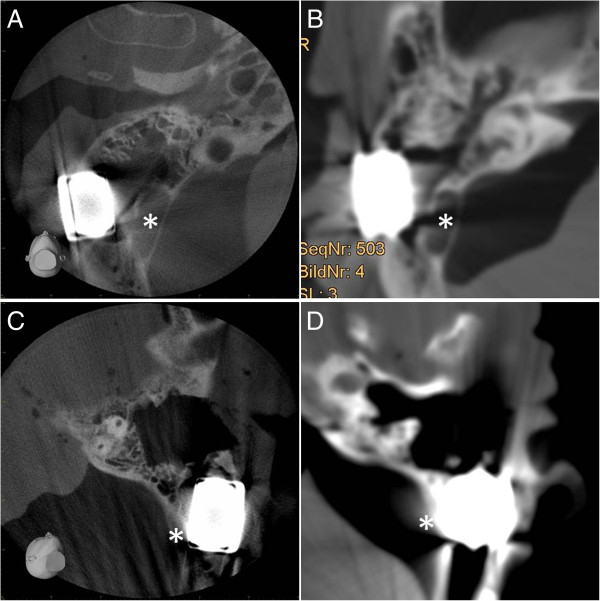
Positional relation of the implant to the sinus sigmoideus. Visualisation of the implants in CBCT (A and C) and in CT (B and D) for both prepared skulls (A and B, head 1; C and D, head 2) in relation to the sinus sigmoideus (*).
